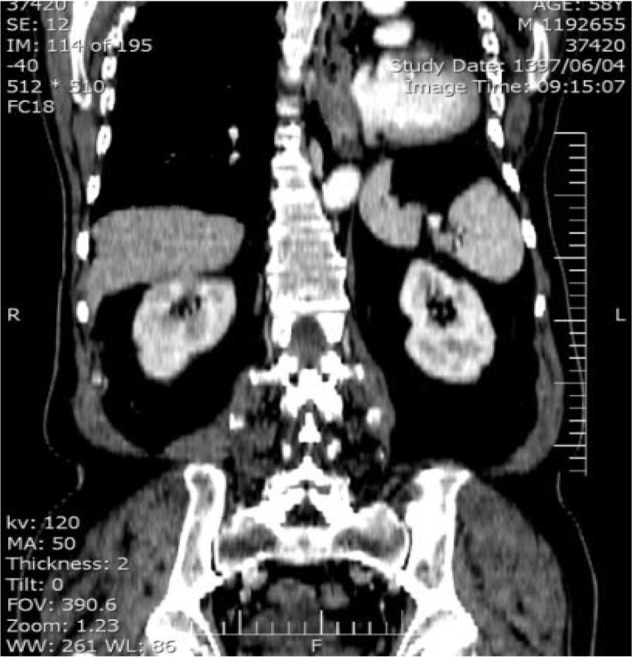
Frontal CT scan, marked deviation after left pneumonectomy.
